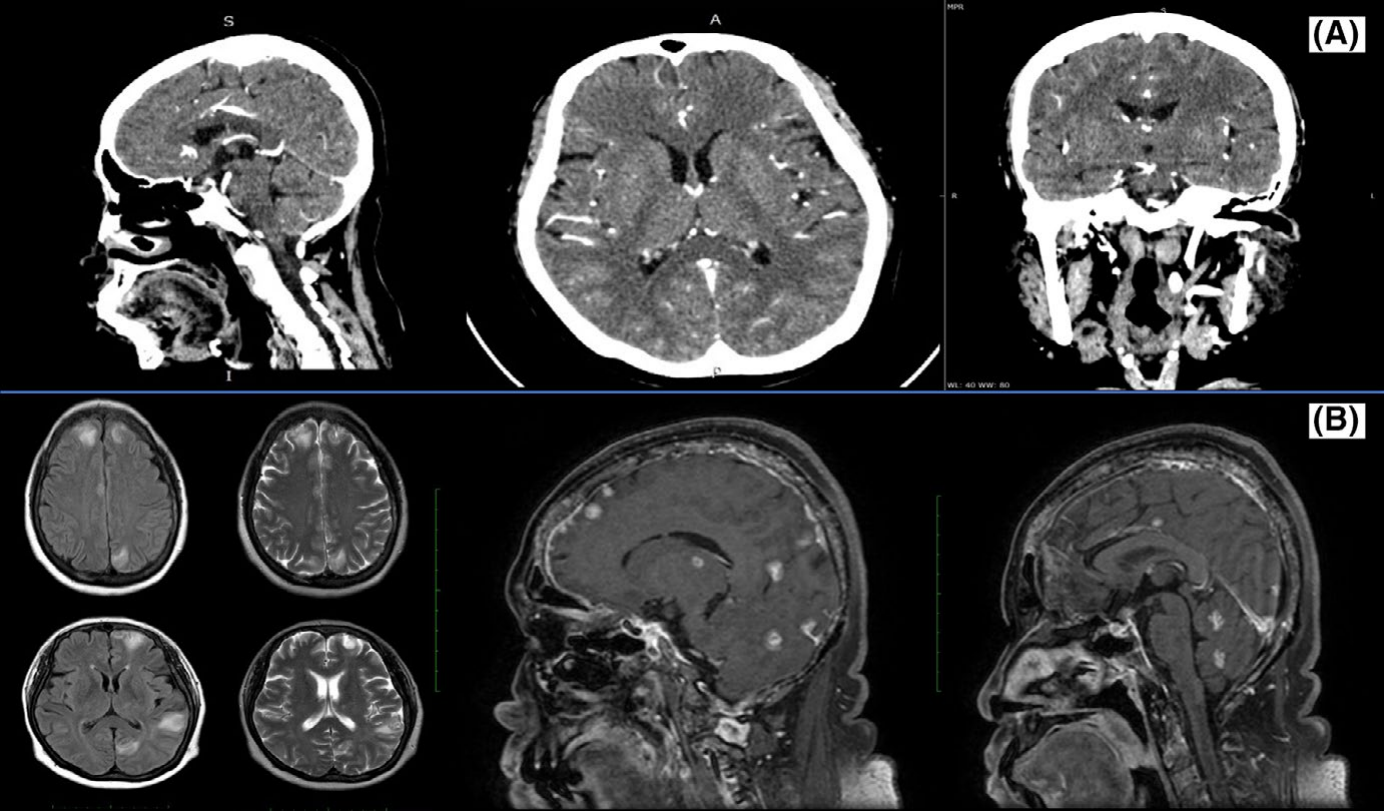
A, Head contrast‐enhanced computed tomography scan in the first seizure episode revealed cortical edema without any sign of metastasis. B, Cerebral magnetic resonance imaging in the second seizure episode showed multiple brain metastases
